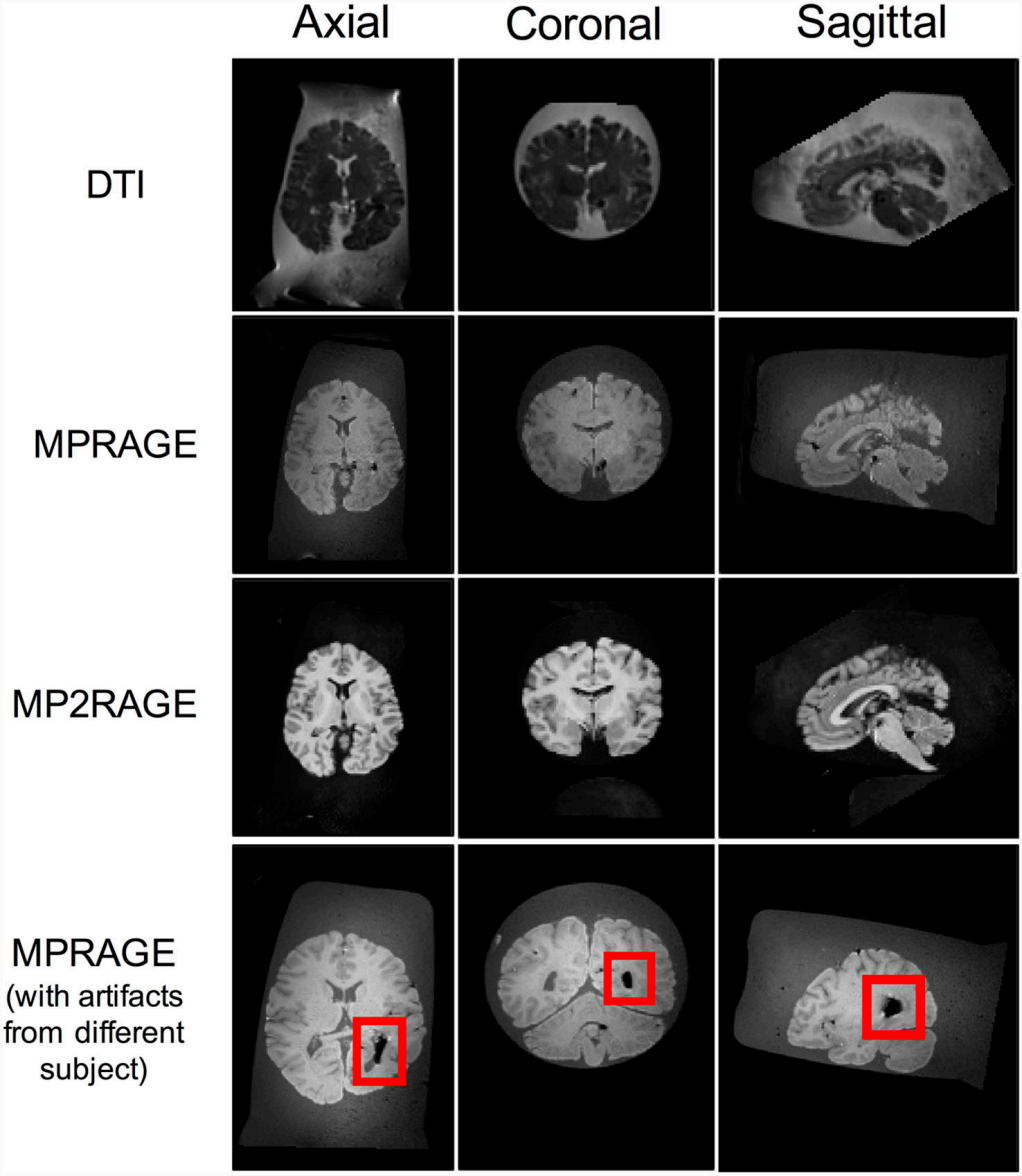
Axial, coronal, and sagittal slice views of a 71-year-old female brain acquired using the aforementioned MRI sequences following the protocols of this report. The image artifact (shown in red box) was caused by air bubbles inside the ventricle.
